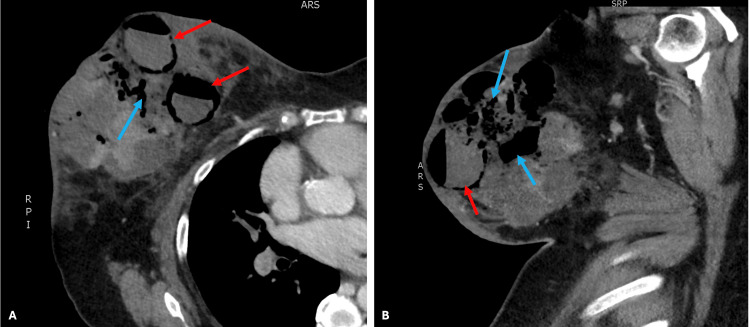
Computed tomography images of the chest showing the right breast: note is made of multiple gas- and fluid-filled collections (red arrows), and subcutaneous gas (blue arrows) A: axial section, B: sagittal section
